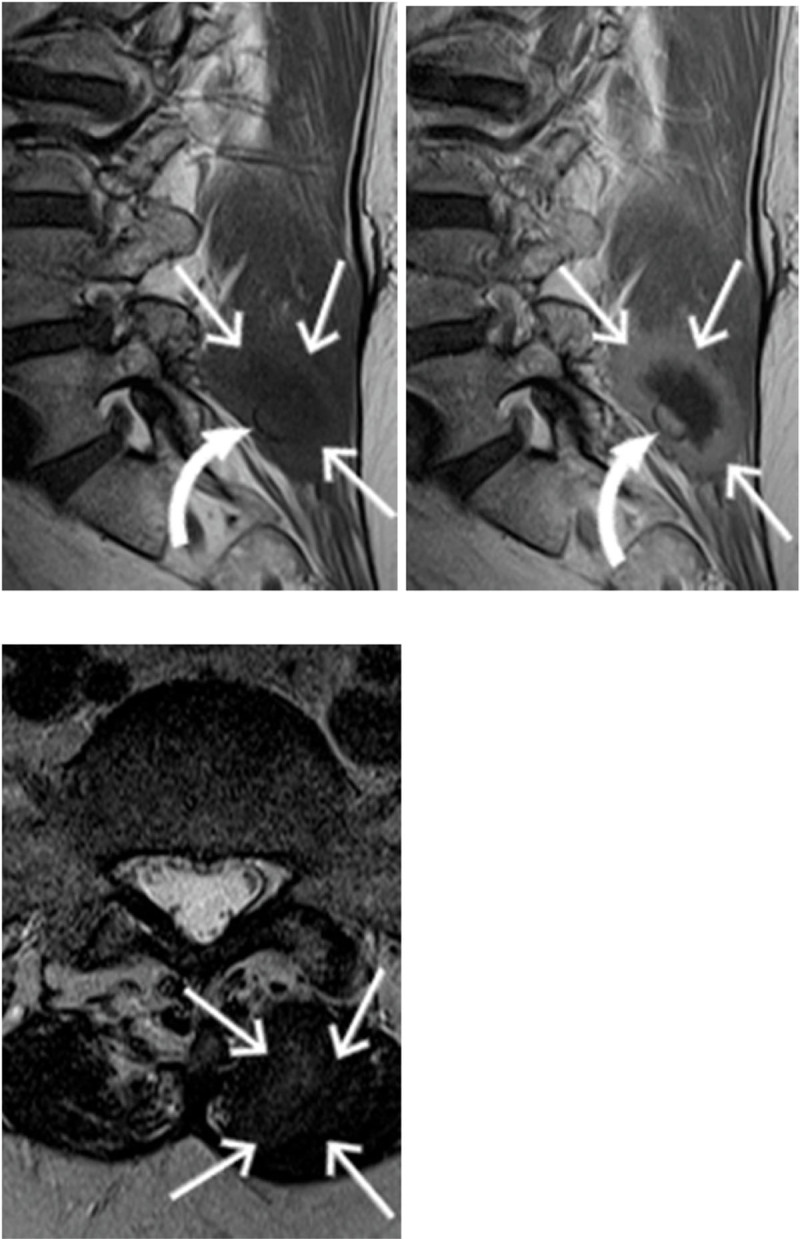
Sagittal T1-weighted MR images before and after intravenous contrast administration. Axial T2-weighted image. Paraspinal mass (arrows) at fourteen months postoperatively. Center of the mass has become hypointense on T2 and the enhancing peripheral wall has thickened. The discovery of a barium sulfate filament (curved arrows) was the characteristic finding to evocate the diagnosis of gossypiboma.
